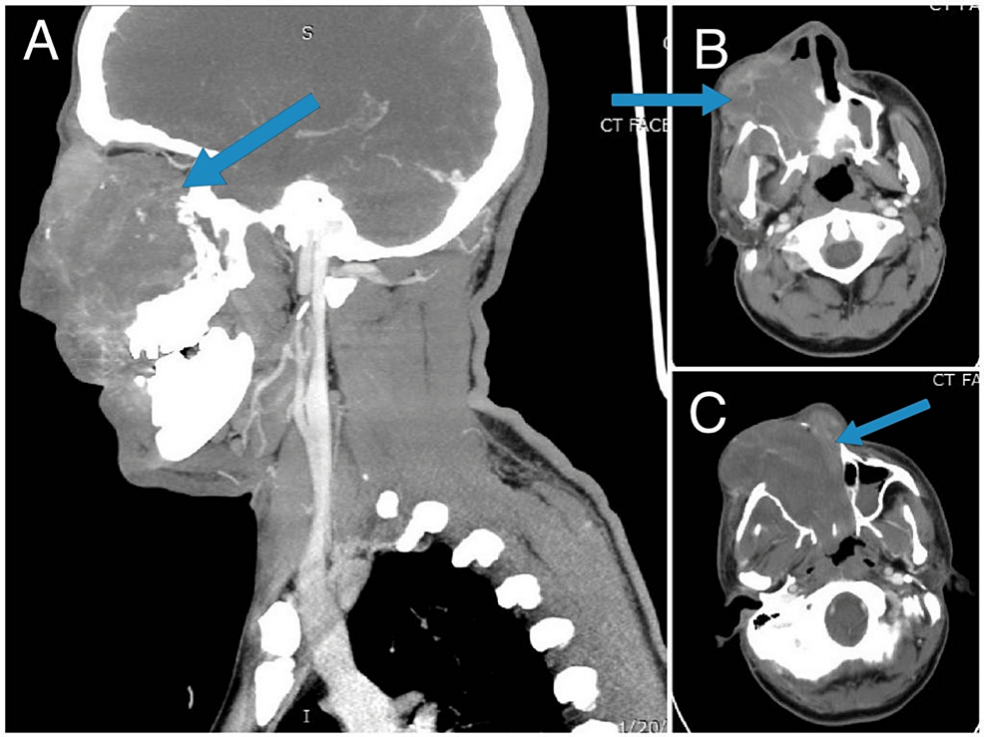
The arrows in the sagittal section in (A) and axial sections in (B) and (C) of CT scan post-re-resection show large soft tissue mass in the right maxillary sinus with pterygopalatine fossa and intraorbital extension. It also invades ethmoidal sinuses and nasal cavity with extensive right-sided neck level IIB lymphadenopathy
